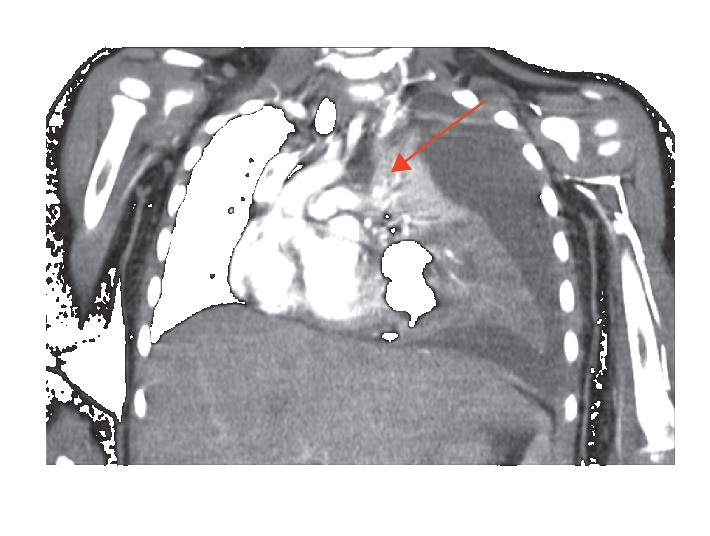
Contrast-enhanced CT of the chest (coronal view) demonstrating mediastinal shift to the right.
